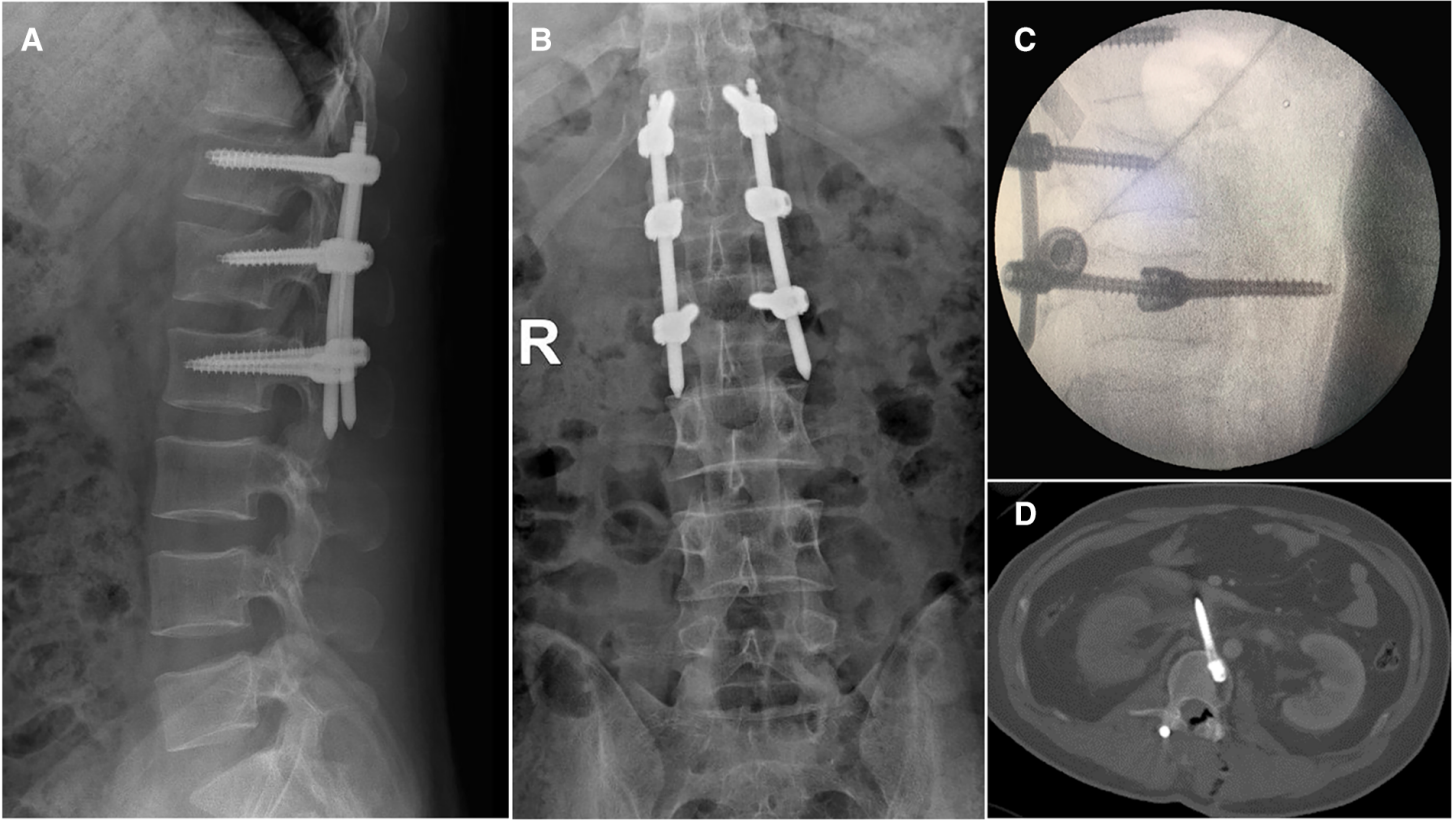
(A,B) anterior-posterior and lateral radiographs 1 year after percutaneous pedicle screw in L1. (C) Intraoperative fluoroscopy was taken and confirmed that the screw migrated anteriorly to the vertebral body. (D) Enhanced CT scanning of the abdomen found that the tip of the right L2 screw indented the IVC and left renal vein without blood extravasation.
